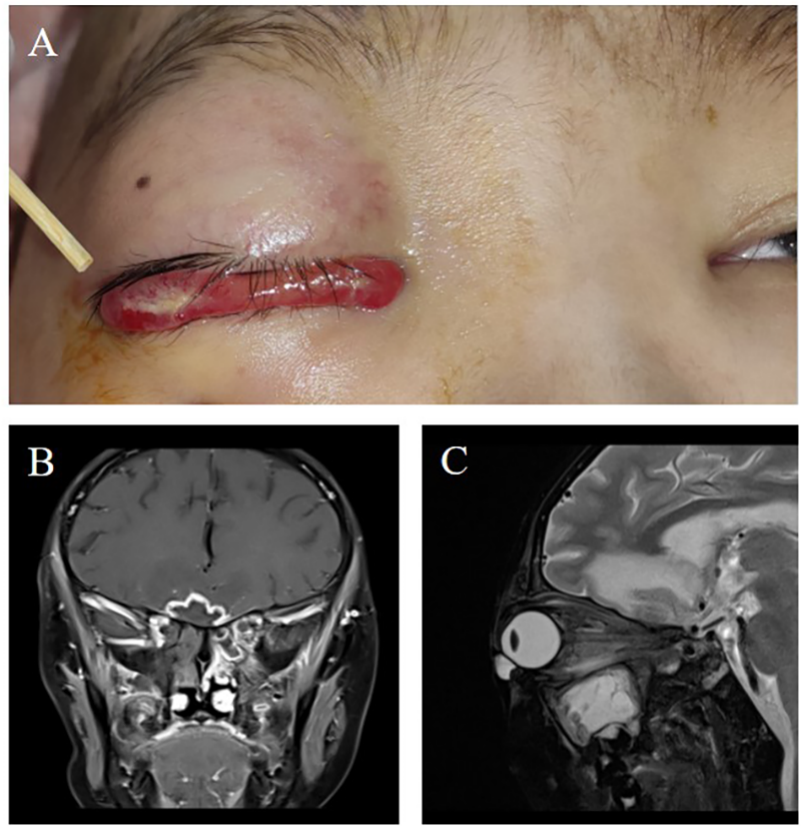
(A) Upon admission, the patient presented with significant redness and swelling in the right eye, accompanied by ptosis of the upper eyelid, impaired vision, and an inability to elevate the eyelid. Antifungal therapy with liposomal amphotericin B and posaconazole was initiated to address the suspected fungal infection. (B) Coronal view and (C) axial view of contrast-enhanced magnetic resonance imaging of the head showed bilateral frontal lobe parenchymal edema with abnormal signal intensities, indicative of a potential infectious process.
